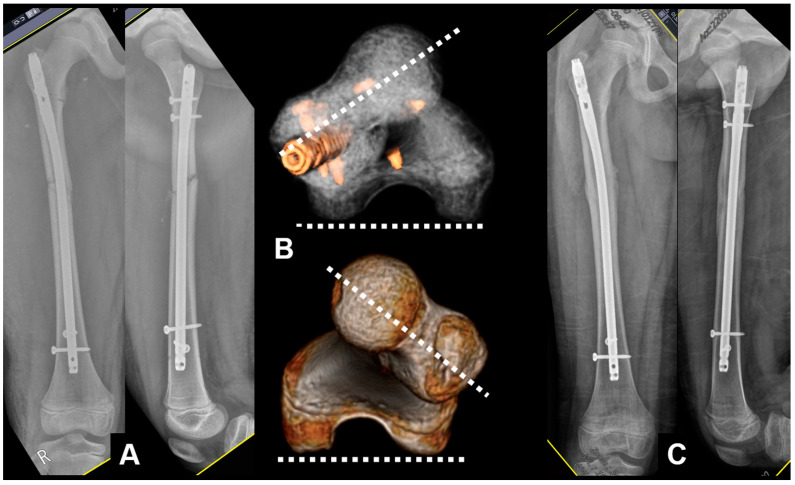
(A) Postoperative radiographs showing revised fixation of distal interlocking; (B) CT scan showing similar angles of anteversion; (C) complete healing observed 6 months postoperatively.
